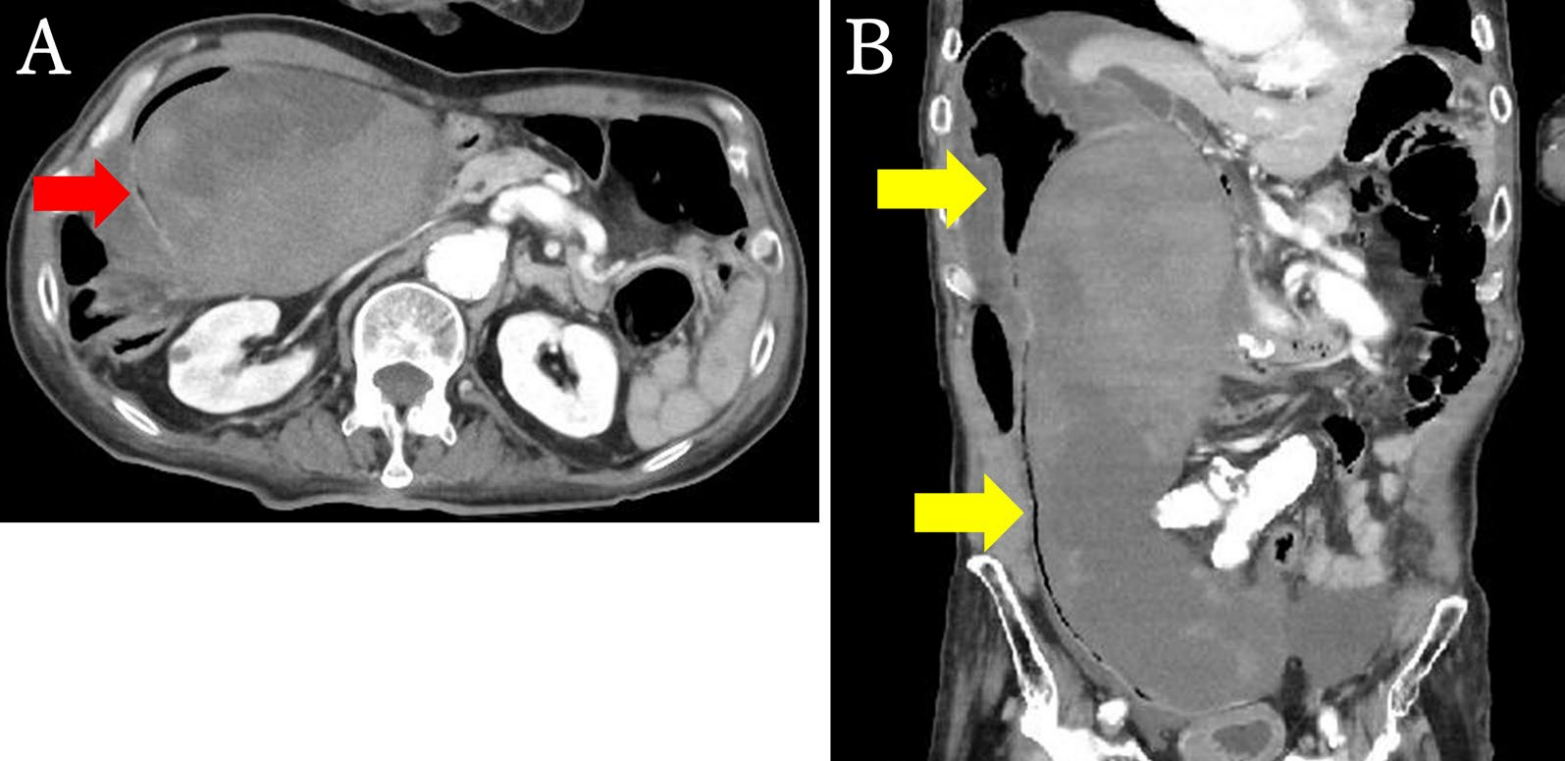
Abdominal contrast-enhanced CT scans. A Cross-sectional image (arterial phase). A 95-mm × 250-mm mass structure of the suspected mesenteric hematoma is observed along the sigmoid colon. The interior is accompanied by a heterogeneous hyperabsorptive zone (red arrow) and the arterial vessels are accompanied by severe atherosclerosis. B Coronal plane image (arterial phase). A mesenteric hematoma is observed along the sigmoid mesentery, compressing the sigmoid colon (yellow arrow). There is no obvious extra aneurysm in or around the hematoma and no free air in the abdominal cavity. CT: computed tomography
